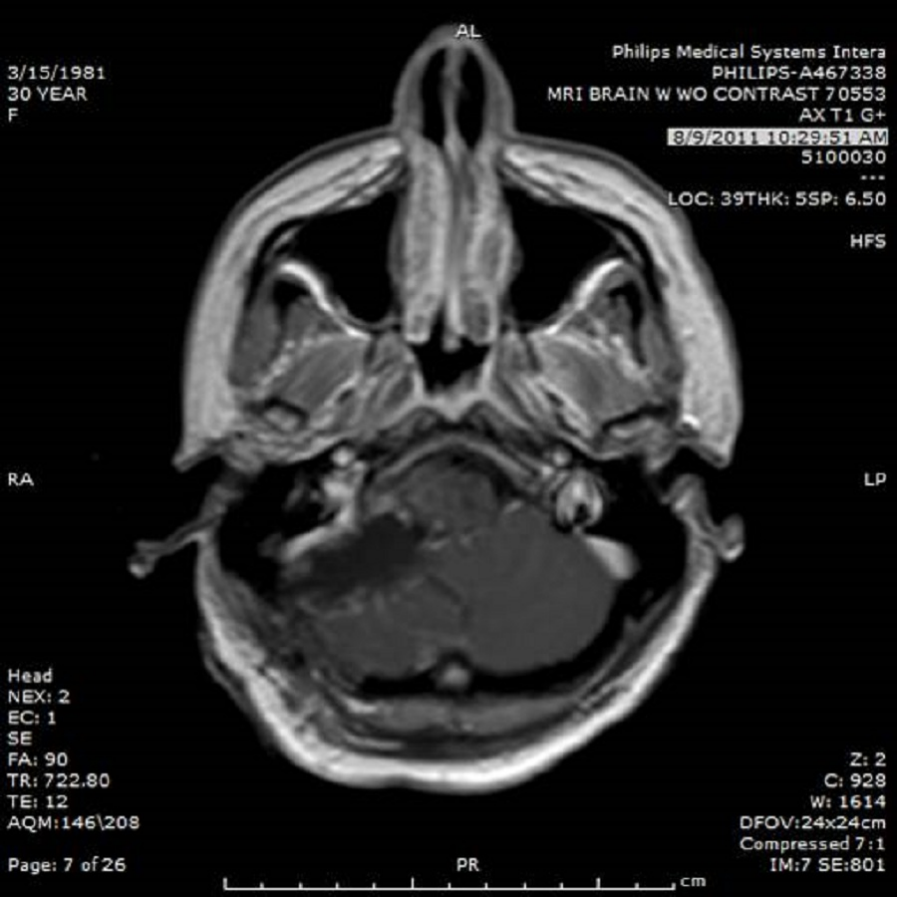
MRI of the brain with contrast detailing the resection cavity postoperatively.
